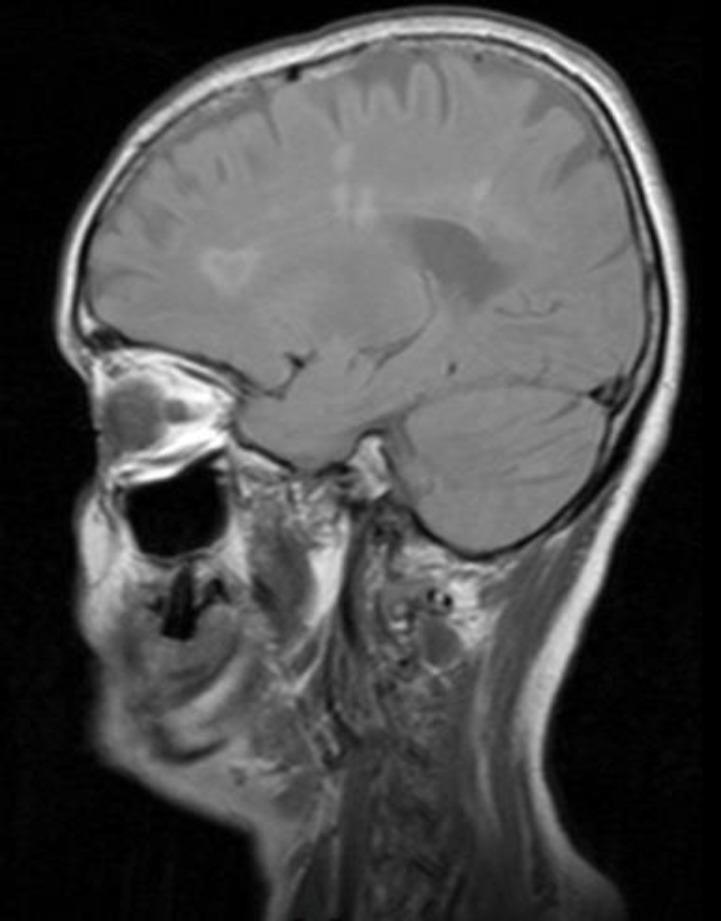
The sagittal FLAIR imaging of the brain MRI showing periventricular hypersignal lesion
